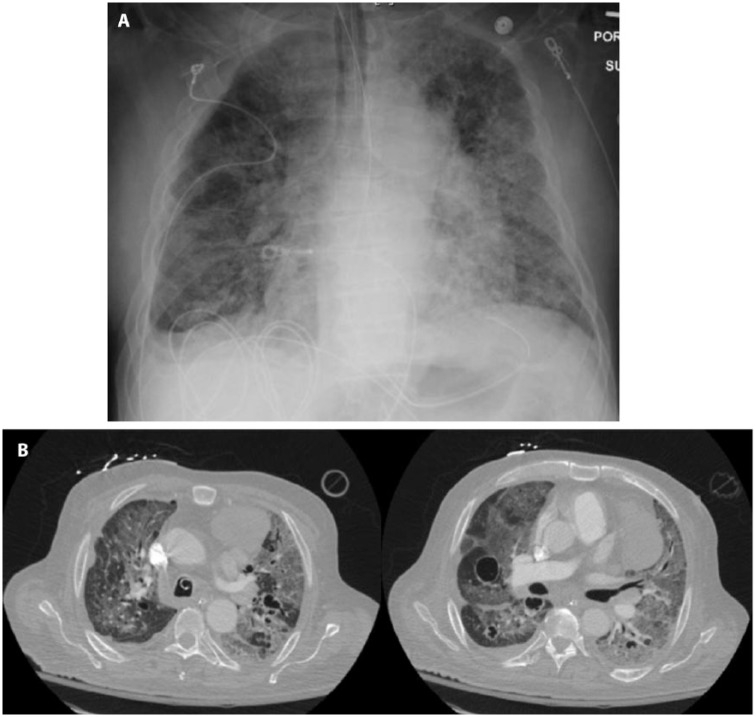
Chest radiographic findings at the time of hospital admission. (A) Chest
